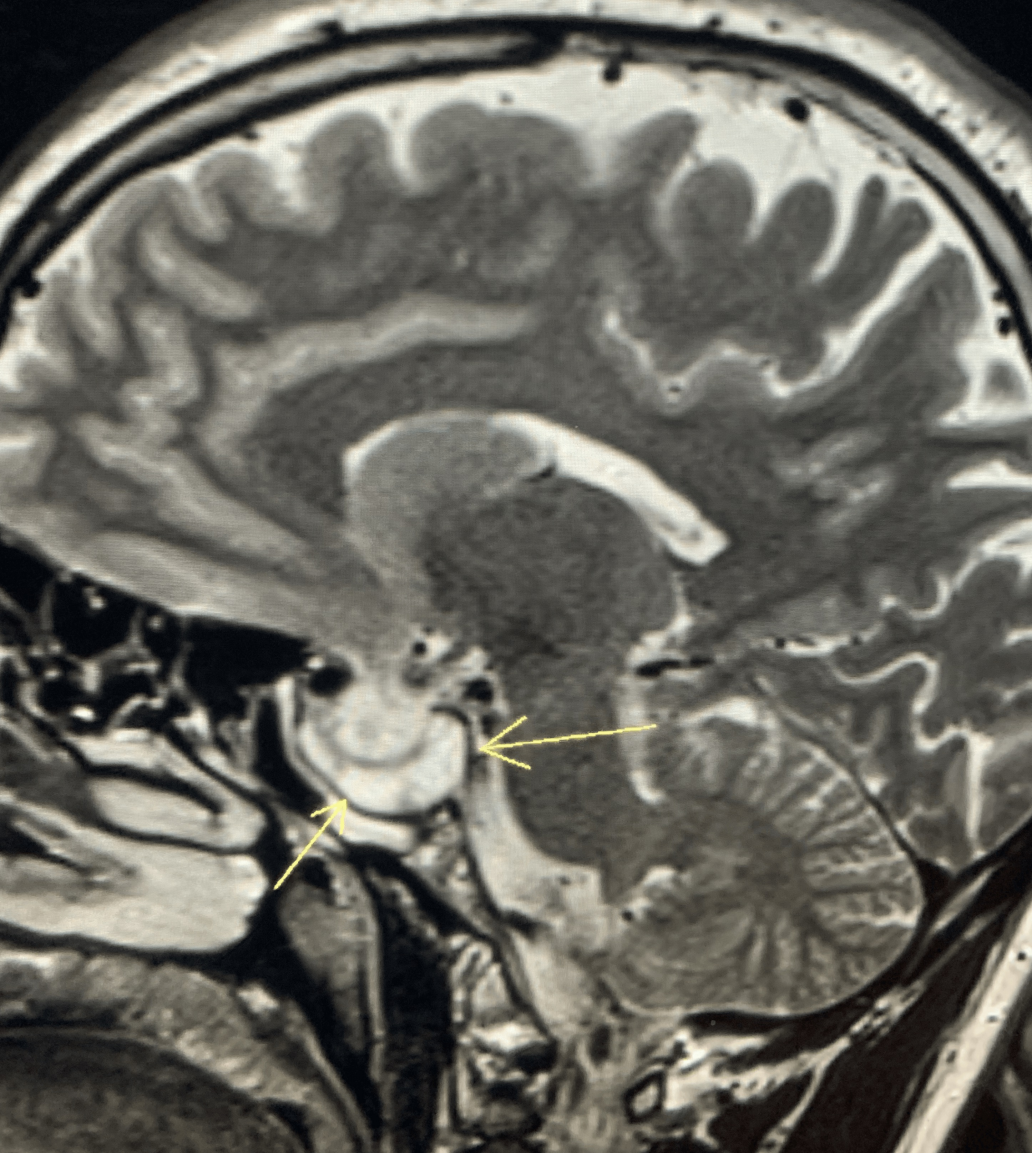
Pituitary MRI - sagittal view Twelve months after treatment.
